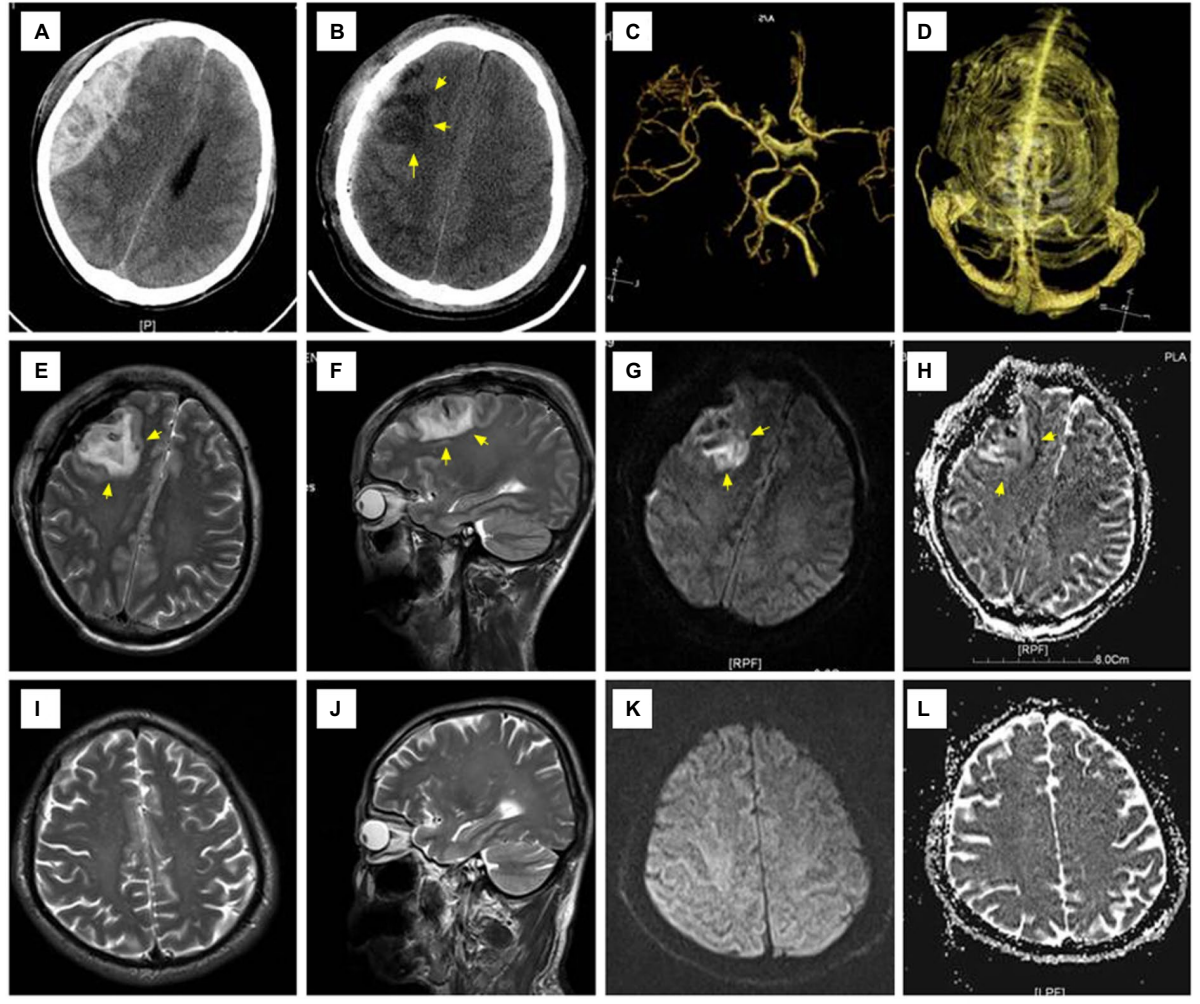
Representative radiographic images of Case 2. (A) An acute epidural hematoma occupying the right frontoparietal area; (B) CT examination performed 3 h after surgery showed patchy cerebral edema mixed with a few spotty areas of hemorrhage in the right frontal lobe; (C,D) CTA and CTV showed no obvious abnormalities; (E–H) T2WI showed a small amount of intracerebral hemorrhage and edema in the right frontal lobe with high signal on both DWI and ADC; (I–L) MRI re-examination 1 month after surgery showed that the lesion disappeared and the involved brain tissue recovered. CTA, CT angiography; CTV, CT venography; T1WI:T1 weighted imaging; DWI, diffusion-weighted imaging; ADC, apparent diffusion coefficient; MRI, magnetic resonance imaging.
